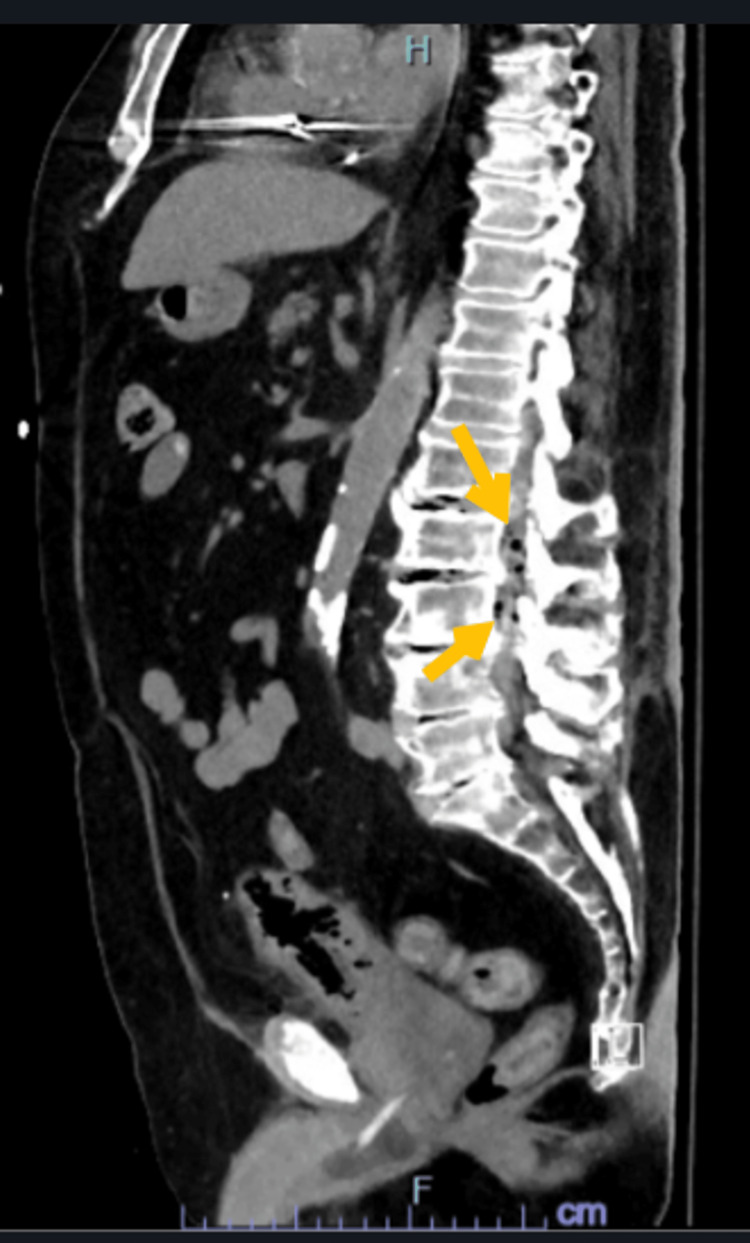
Non-contrast CT of the abdomen and pelvis (sagittal section). Air bubbles extending from the paraspinal muscles into the epidural space of the lumbar spinal canal (arrows). CT: computed tomography
